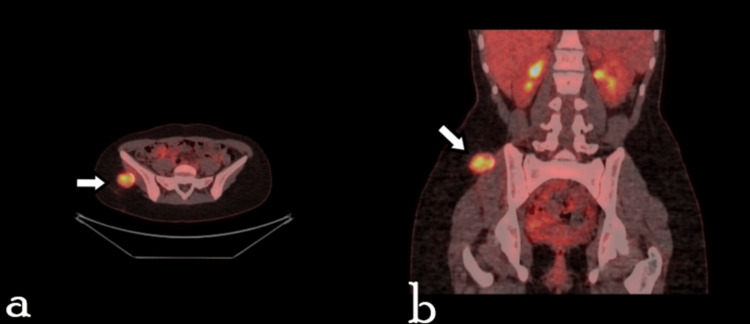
Transverse and coronal 18F-FDG PET/CT images show a hypermetabolic lesion within the adipose tissue, in contact with the gluteal muscle (a) Maximum intensity projection image shows a solitary hypermetabolic lesion within the soft tissues of the right gluteal region, along with axial CT (in bone and tissue windows), PET, and PET/CT sections. (b) The same sections shown in coronal view. The SUVmax of the lesion was 6.6, and the radiodensity measured 42 and 225 HU in the interior and at the calcified rim of the lesion, respectively (for comparison, the radiodensity of the cortical iliac bone was 560 HU).
